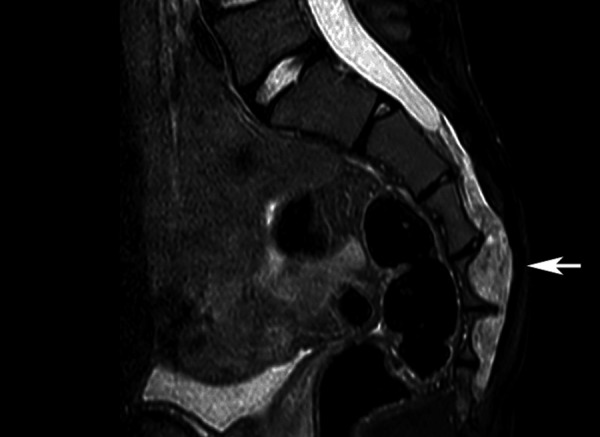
T2-weighted MRI of the extensive extradural tumor at the sacral level.
